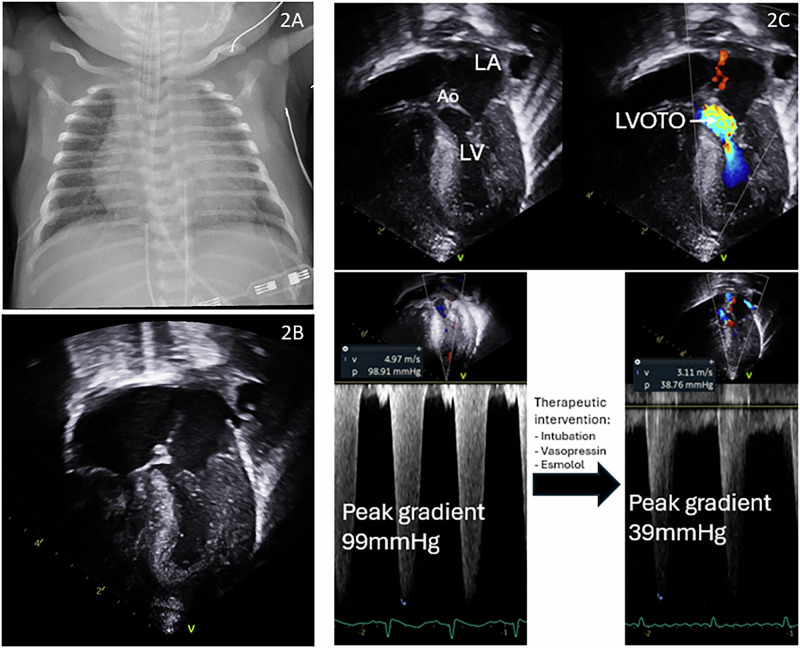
Initial and subsequent imaging showing resolution of cardiac hypertrophy. A Initial chest X-ray showing cardiomegaly. B 2-dimensional echocardiogram showing asymmetrical septal hypertrophy. C Selected echocardiography clips with LVOT gradient pre and post therapeutic interventions. (LA: left atrium; Ao: Aorta; LV: left ventricle; LVOTO: LV outflow tract obstruction).
